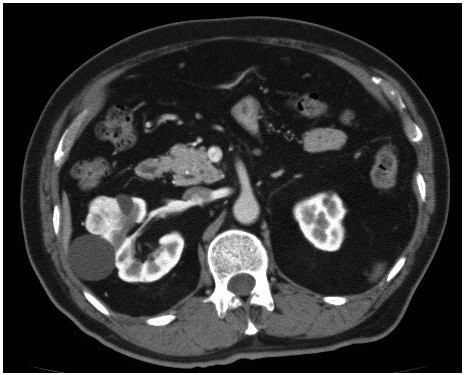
Enhanced abdominal computed tomography revealed a right renal tumor at the upper renal pole.
